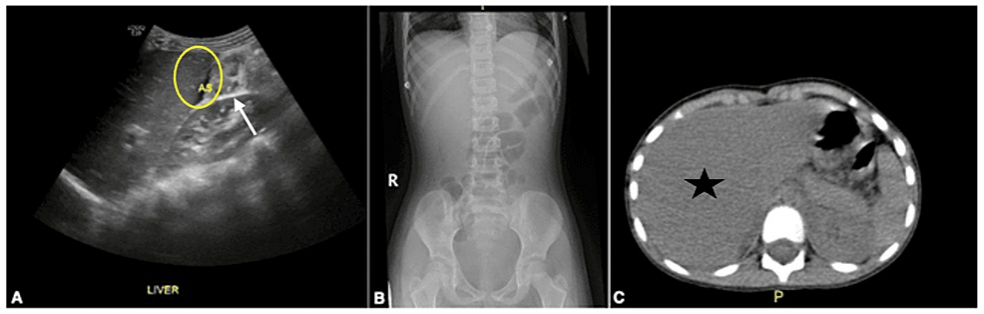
Abdominal US showing small ascites (circle) with edematous gallbladder wall thickening (arrow) (A). Abdominal X-ray showing no significant abnormality (B). CT abdomen with contrast depicting mild liver enlargement (star) and diffuse intestinal wall thickening (C). US: ultrasound; CT: computerized tomography
